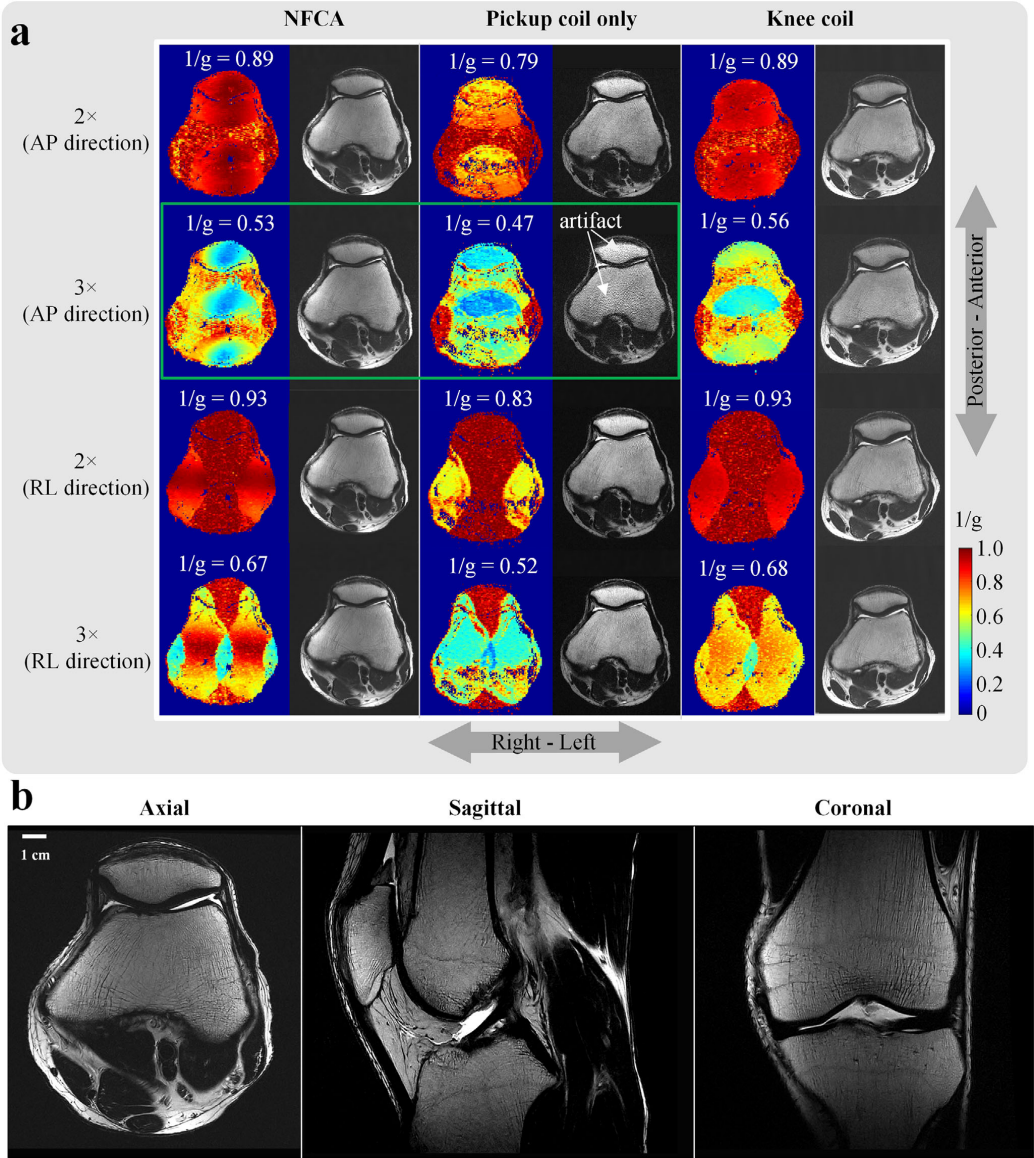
In vivo study. a) Comparison of the parallel imaging capabilities of NFCA, its pickup coil, and the knee coil. 1/g maps under identical acceleration conditions are shown (g represents the mean g‐factor, and higher 1/g values indicate better parallel imaging performance). Next to the 1/g maps are the anatomical images obtained from the MR system's built‐in software. b) The T2‐weighted MRI images of the cross‐sectional, sagittal, and coronal planes obtained using NFCA have a high resolution of 0.33 mm × 0.33 mm × 2 mm.
